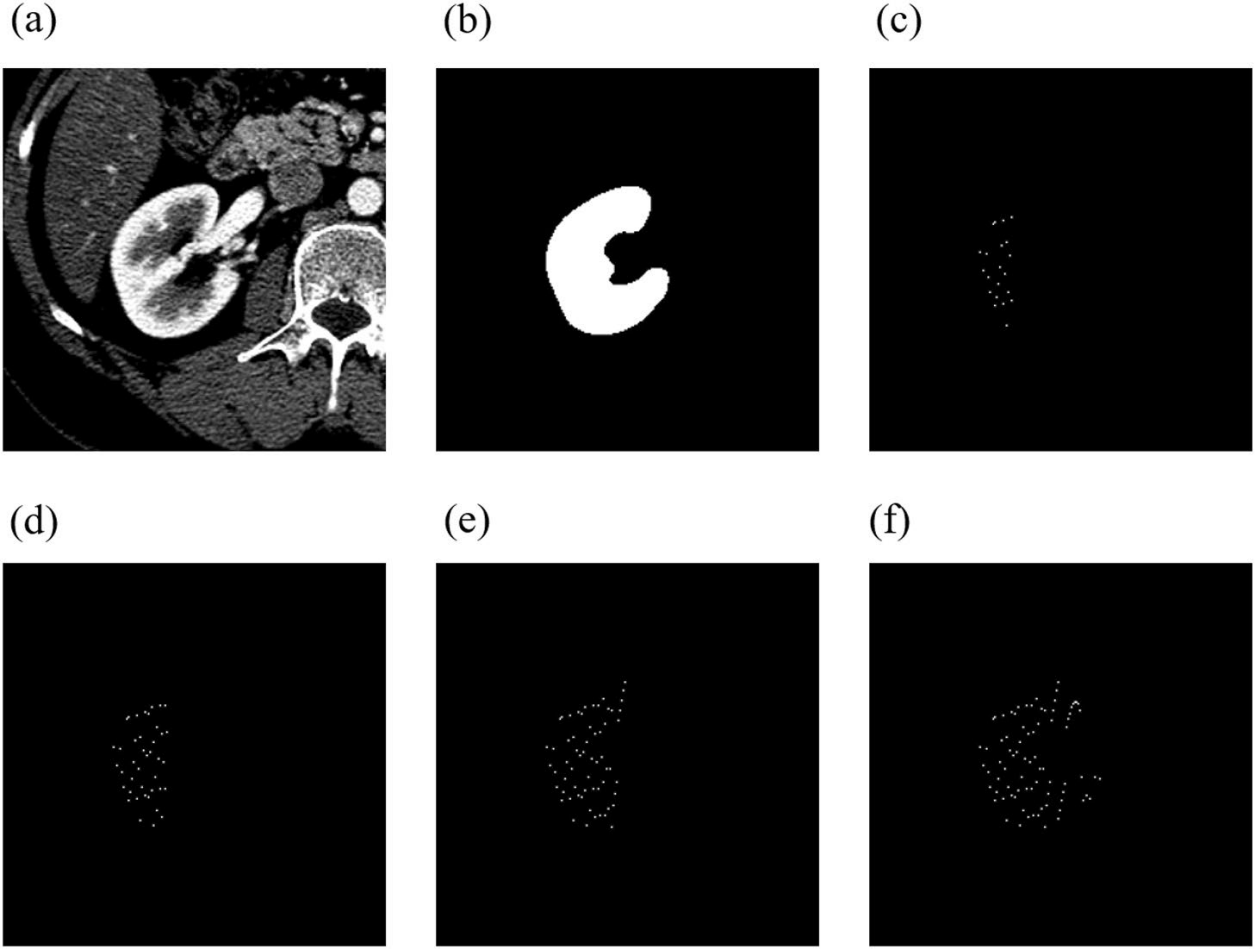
The process of generating points for the class OC. (a) shows a fragment of the original CT image. (b) shows a mask with a kidney labelled by an expert. (c–f) shows the point generation process.
